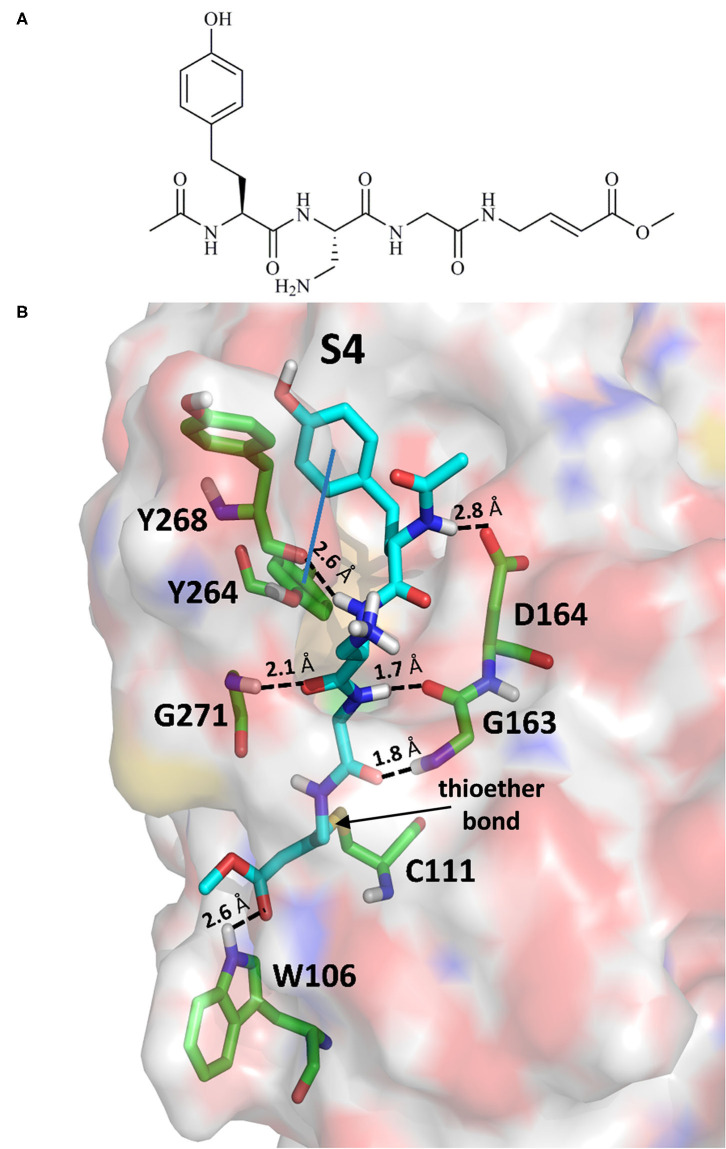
(A) 2D sketch of VIR251; (B) X-ray coordinates of VIR251 within the PLpro binding site (PDB ID 6WX4). VIR251 and important residues are rendered as sticks, whereas the protein is represented as a surface. H-bonds are represented by dotted black lines, whereas the pi-stacking interaction between VIR251 and Y264 is itemized by a blue line. For the sake of clarity, only polar hydrogen atoms are shown.
